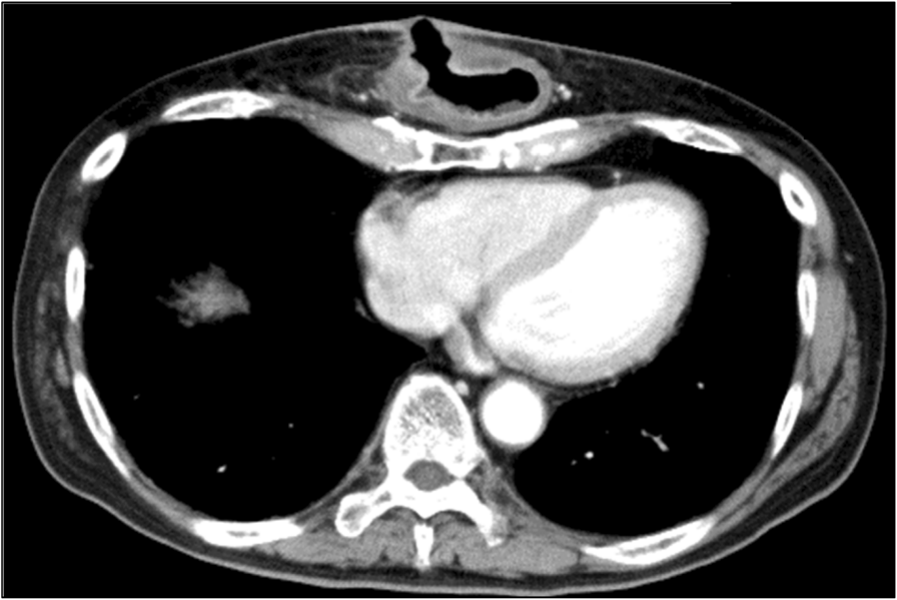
Chest CT showed a fistula between the gastric conduit and precordial skin
